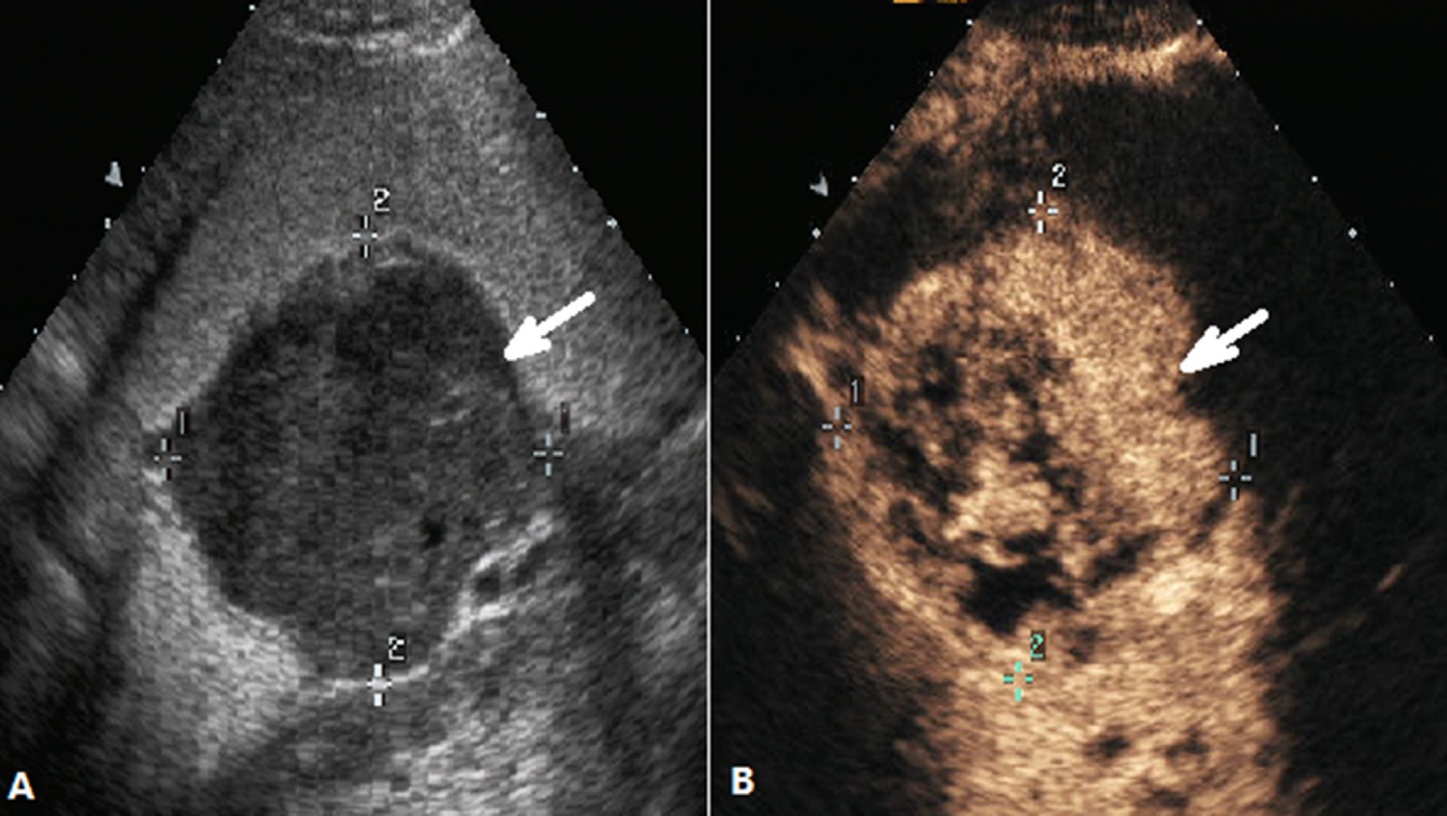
DCEUS images of a case classified as Borrmann type I (A) Ultrasonography showed the thickened gastric wall with nodular polypoid appearance(arrow) after the stamoch was filled with UOCA, (B) The same view showed that the lesion(arrow) was enhanced after administration of intravenous contrast agent.
